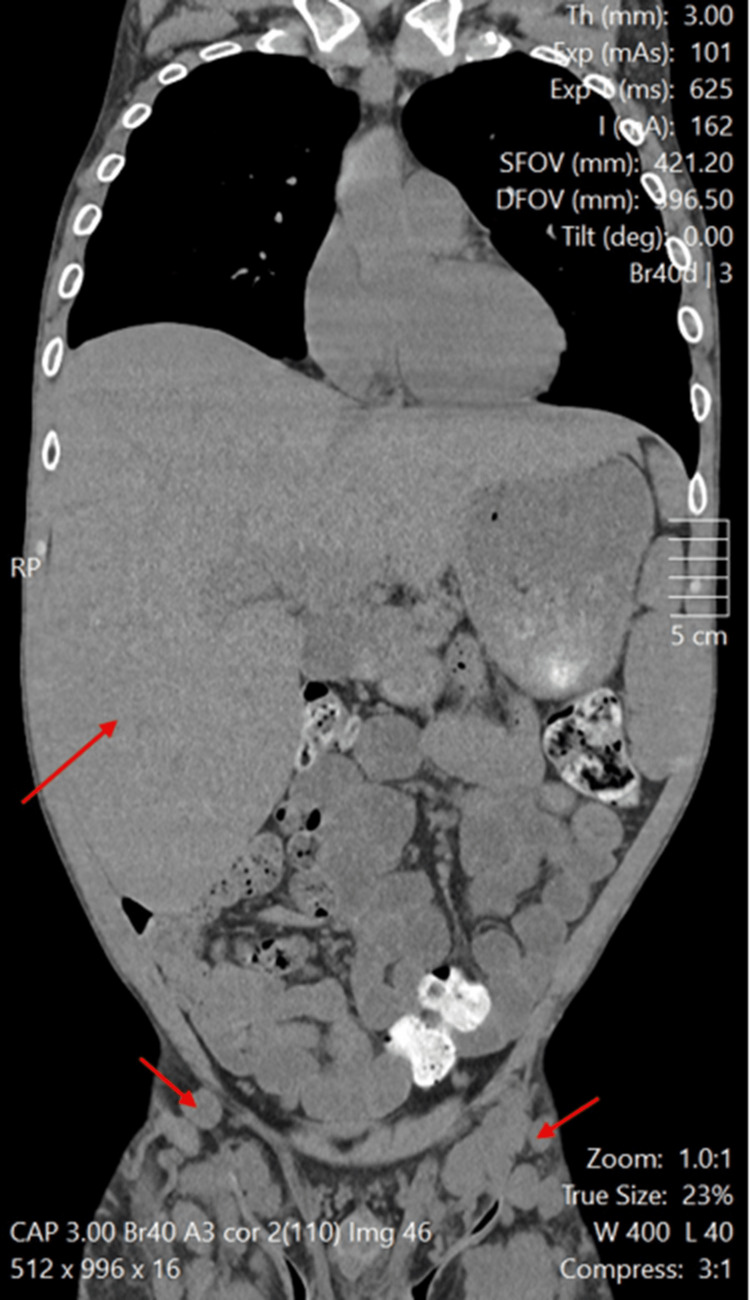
Coronal CT scan showing marked hepatosplenomegaly and inguinal lymphadenopathy
